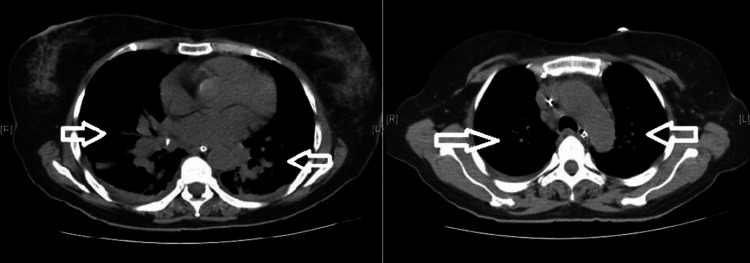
CT chest revealing enlarged lymph nodes in the pre-carinal, pre-tracheal, and subcarinal regions CT: computed tomography
